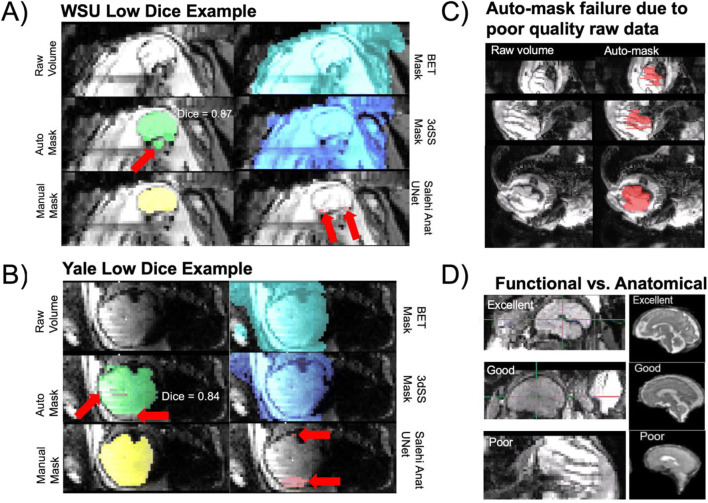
Failure analysis and comparison of functional with structural fetal MRI data. (A) All data of the WSU test set subject with the lowest Dice coefficient (0.87). (B) All data of the Yale test set subject with the lowest Dice coefficient (0.84). The BET, 3dSkullStrip, and Anatomical U-Net masks do not adequately capture the fetal brain’s boundary in both the WSU and Yale case. (C) Extreme failure of the auto-mask model, due to very poor quality of the raw data. (D) Comparison of data quality between fetal functional and structural MRI data to understand why models designed for brain segmentation of anatomical data do not necessarily translate to functional data. Structural fetal MRI image used with permission from Payette et al. (2021)
